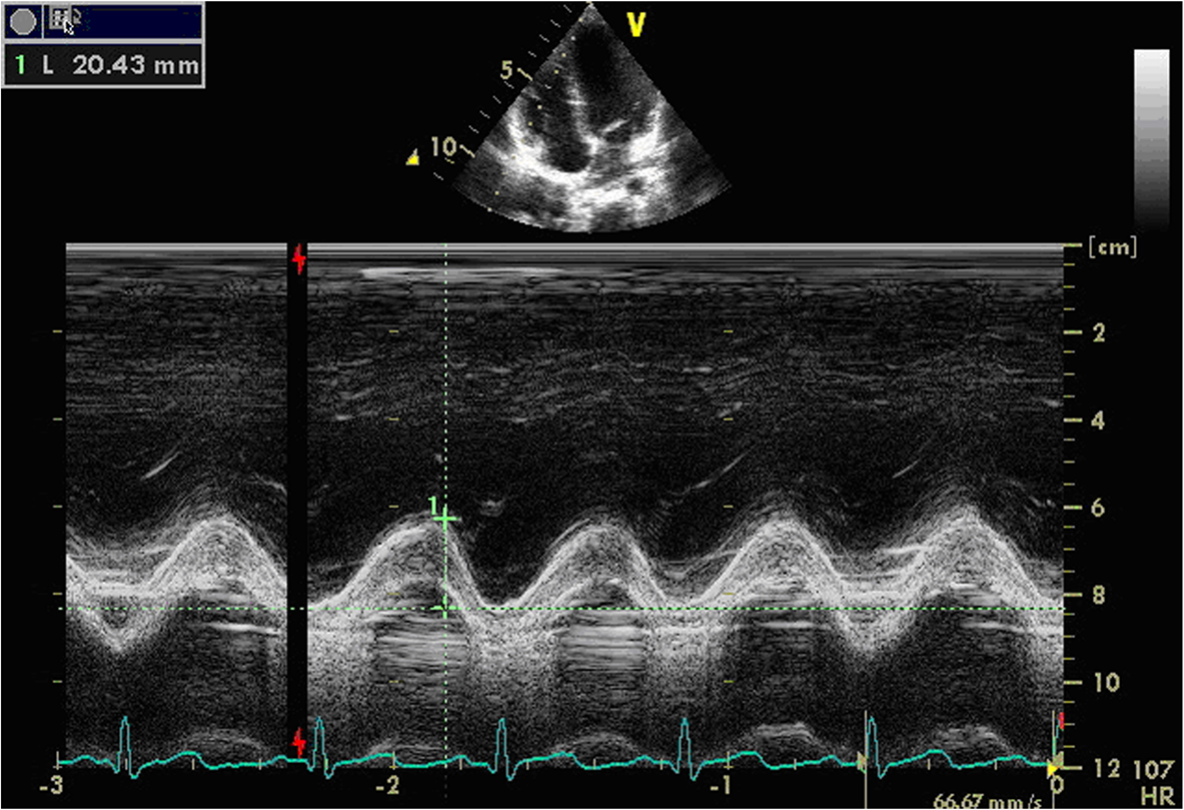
TAD measured from the RV lateral wall (TADl). The upper image was the apical 4-chamber view, the lower curve was the M-mode line of RV lateral wall. TADl was the distance measured form the highest to the lowest of the line (in the above image).
